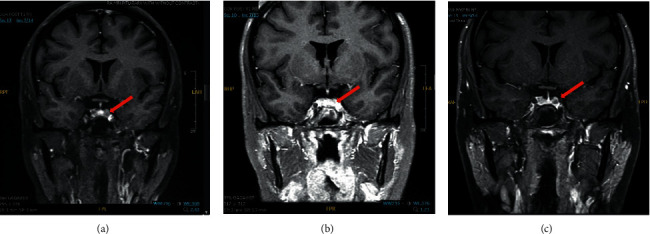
(a) Initial: MRI pituitary with and without contrast showing a coronal T1 postcontrast image immediately prior to our patient's pituitary surgery. The red arrow points to a 3 × 3 × 5 mm hypoenhancing focus representing a pituitary microadenoma. (b) Postsurgical: MRI pituitary with and without contrast showing a coronal T1 postcontrast image obtained three months after transsphenoidal pituitary surgery. The red arrow shows that a hypoenhancing focus is no longer seen and has been resected. (c) Postpartum: MRI pituitary with and without contrast showing a coronal T1 postcontrast image obtained four weeks postpartum. The red arrow points to a 3 mm relatively hypoenhancing lesion representing a recurrent pituitary adenoma.
